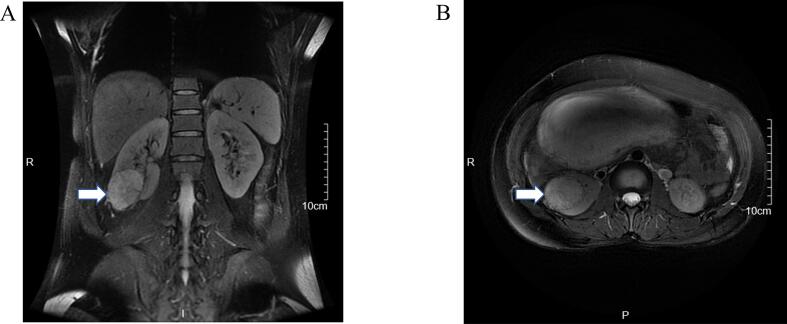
MRI demonstrated a 4.6 × 4.1 × 5.3 cm mass at the lower pole of the right kidney, exhibiting heterogeneous signal intensity on T2. Coronal (A) and Axial (B). The white arrow indicates the tumor.
